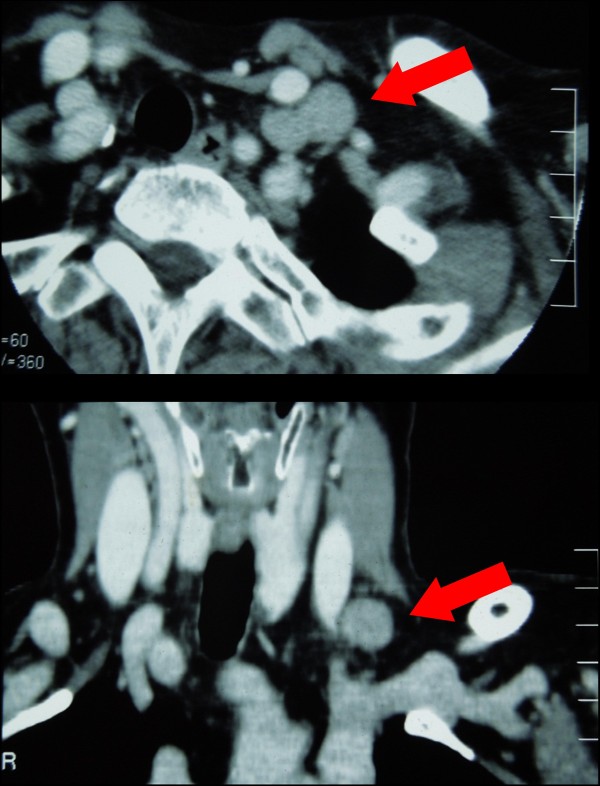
Preoperative CT findings. The CT showed a single left supraclavicular lymph node (arrow) (a) axial section, (b) coronal section
